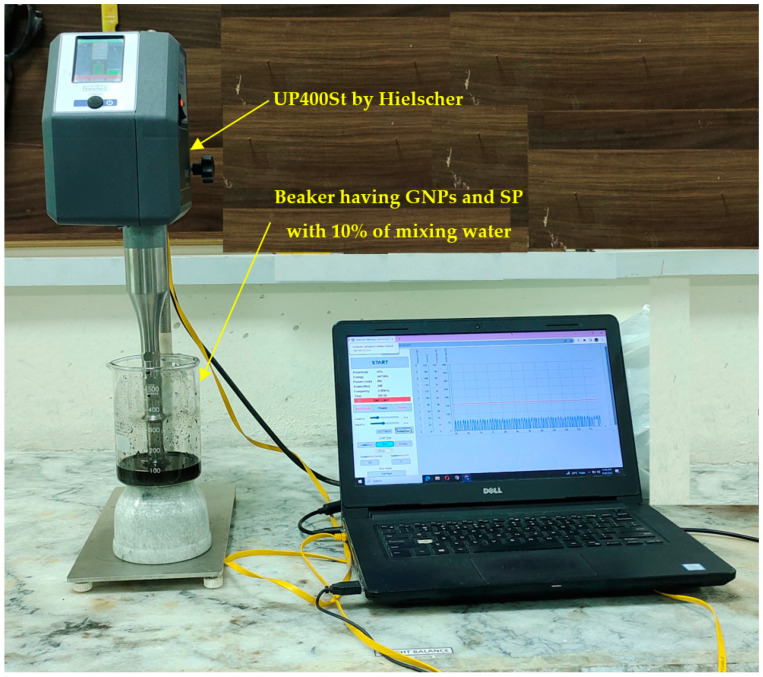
Application of UP400St ultrasonicator, Hielscher, Germany for ultrasonication.
